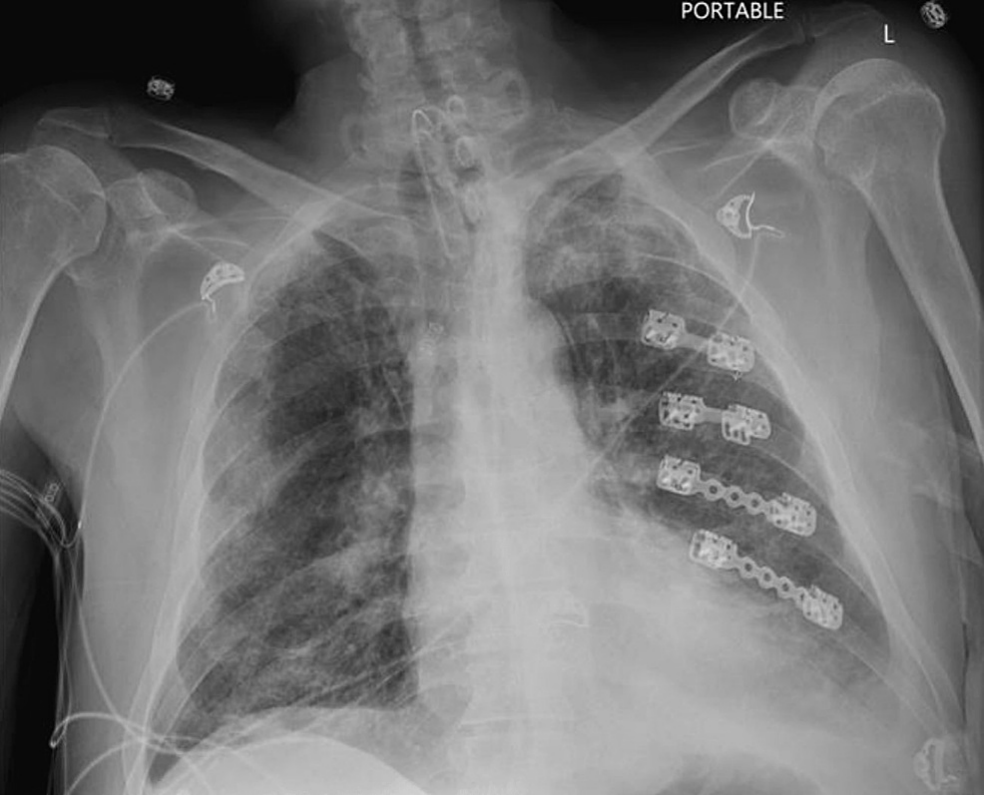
Admission chest x-ray Prominent bilateral interstitial lung marking with partial consolidation of the left lower lobe with effacement of the left hemidiaphragm and retrocardiac region. Multiple right and left rib cage fractures; status post open reduction and internal fixation of the left fifth through left eighth ribs.
